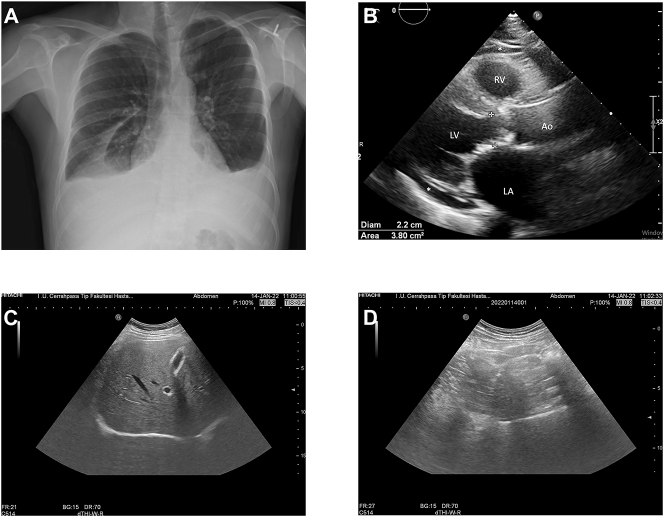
The chest X-ray, transthoracic echocardiography, and abdominal ultrasonography images of Patient #4. (A) Bilateral pleural effusion in chest X-ray. (B) Minimal pericardial effusion detected in TTE (Ao, aorta; LA, left atrium; LV, left ventricle; RV, right ventricle. *Parasternal long-axis view demonstrating pericardial effusion). (C and D) AUS showing no ascites in the perihepatic area (C) and no peritoneal fluid between the bowel loops (D).
